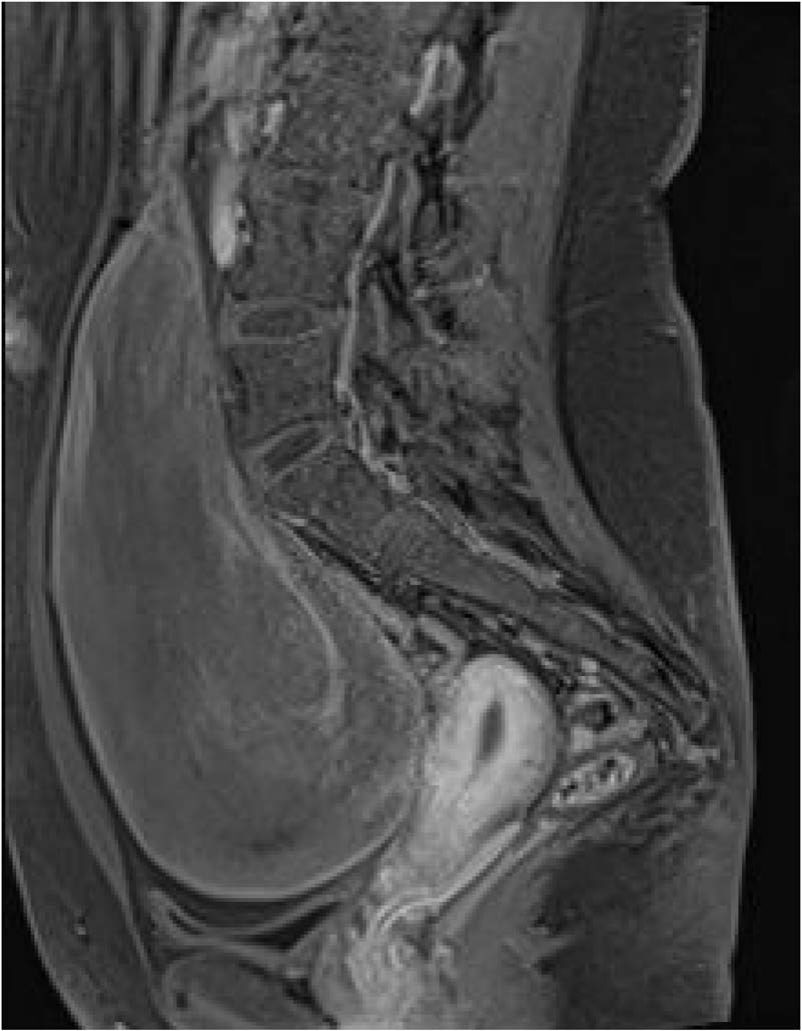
MRI abdomen and pelvis with contrast (sagittal view) demonstrating a 9.3 × 15.3 × 18.9 cm well defined, solid space occupying lesion displacing the urinary bladder inferiorly and the uterus and ovaries into the pre-sacral area.
